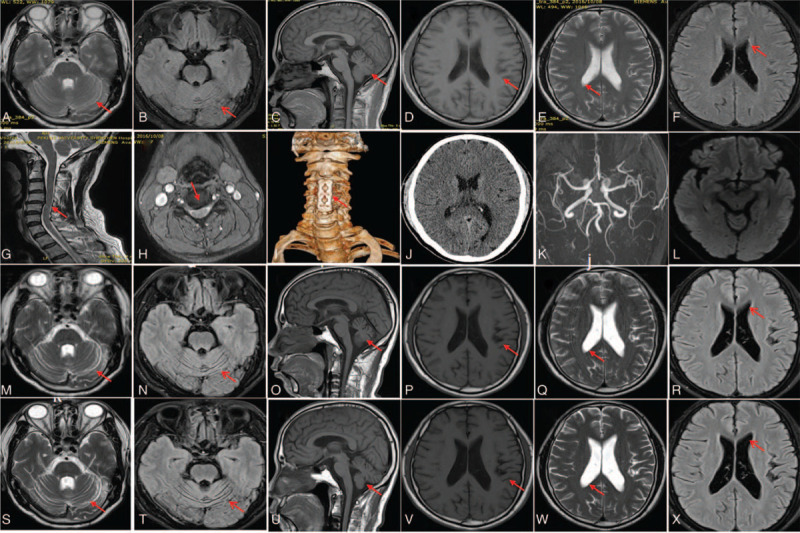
(A–F) Magnetic resonance imaging (MRI) (the sequences are T2-weighted imaging [WI], fluid-attenuated inversion recovery [FLAIR], T1-WI, T1-WI, T2-WI, and FLAIR, respectively) shows no obvious abnormality in October 2016. (G–H) Cervical MRI shows cervical disc herniation at cervical 3/4 (C3/4), C4/5, and C5/6 (arrow) and compression of spinal compression at C4/5 level (arrow). (I) Postoperative cervical vertebra computed tomography three-dimensional imaging shows postoperative changes (arrow) of C3-5 vertebral body and intervertebral space. (J) Brain computed tomography scan shows no obvious abnormality in April 2017. (K–L) Brain magnetic resonance angiography (k) and diffusion WI (l) show no obvious abnormality in February 2019. (M–R) Relatively deep sulcuses in the cerebellar hemisphere (m-o, arrows), insular cortex (p, arrow), and lateral ventricle enlargement (q-r, arrows) on MRI having an aggravating trend in February 2019. (S–X) MRI shows aggravation of cerebral and cerebellar atrophy (arrows) in May 2020 compared with MRI in February 2019.
